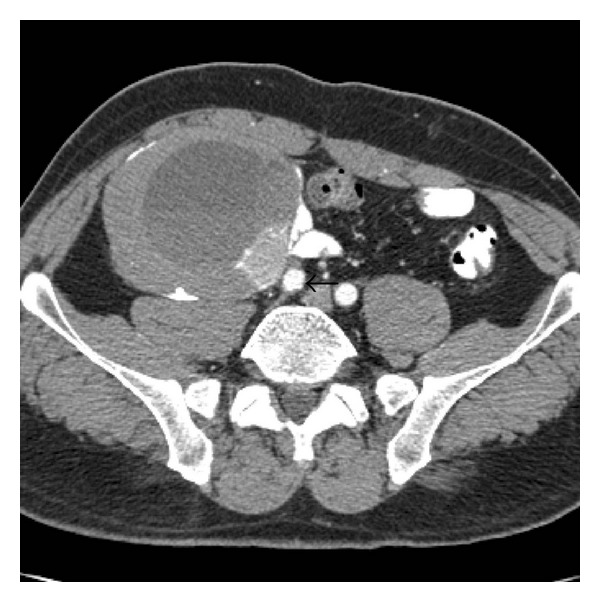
On enhanced CT, the wall and septa were not enhanced.
